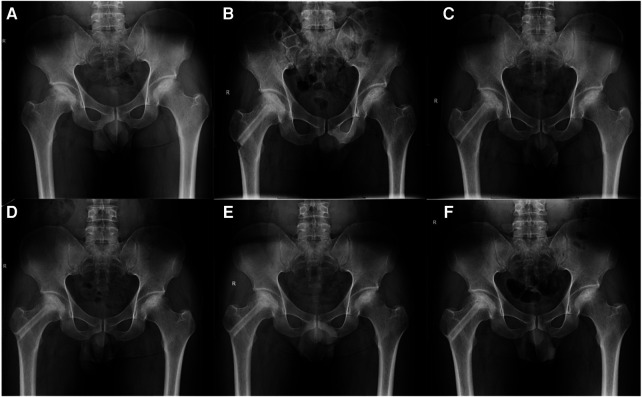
A 39-year-old male patient with alcohol-related ONFH on the right hip. (A) Pre-operative radiograph. (B) Non-vascularized bone was grafted at post-operative radiograph. (C–E) The necrotic area is being repaired by the support of allograft at 3, 6, and 12 months post-operatively. (F) Follow-up radiography at 33 months post-operatively showing no radiography progress on his right hip.
